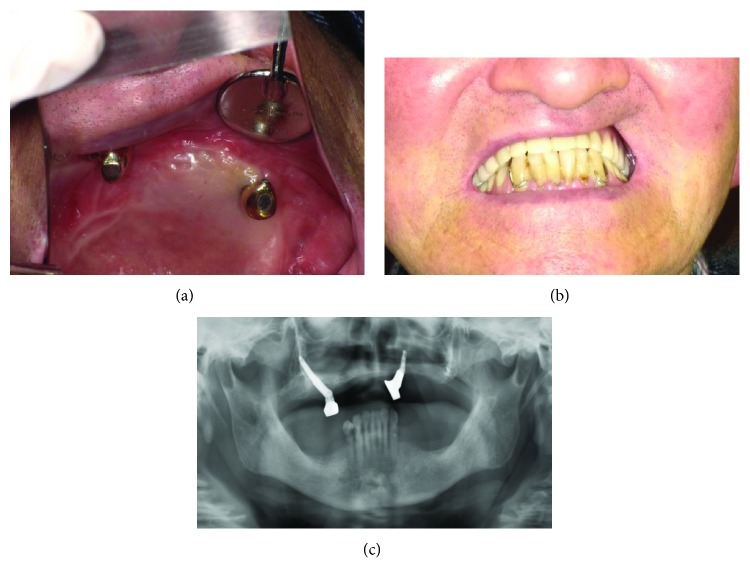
(a) Postoperative intraoral photograph (mirror image). (b) Intraoral view with the prosthesis in place. (c) Postoperative radiograph.
